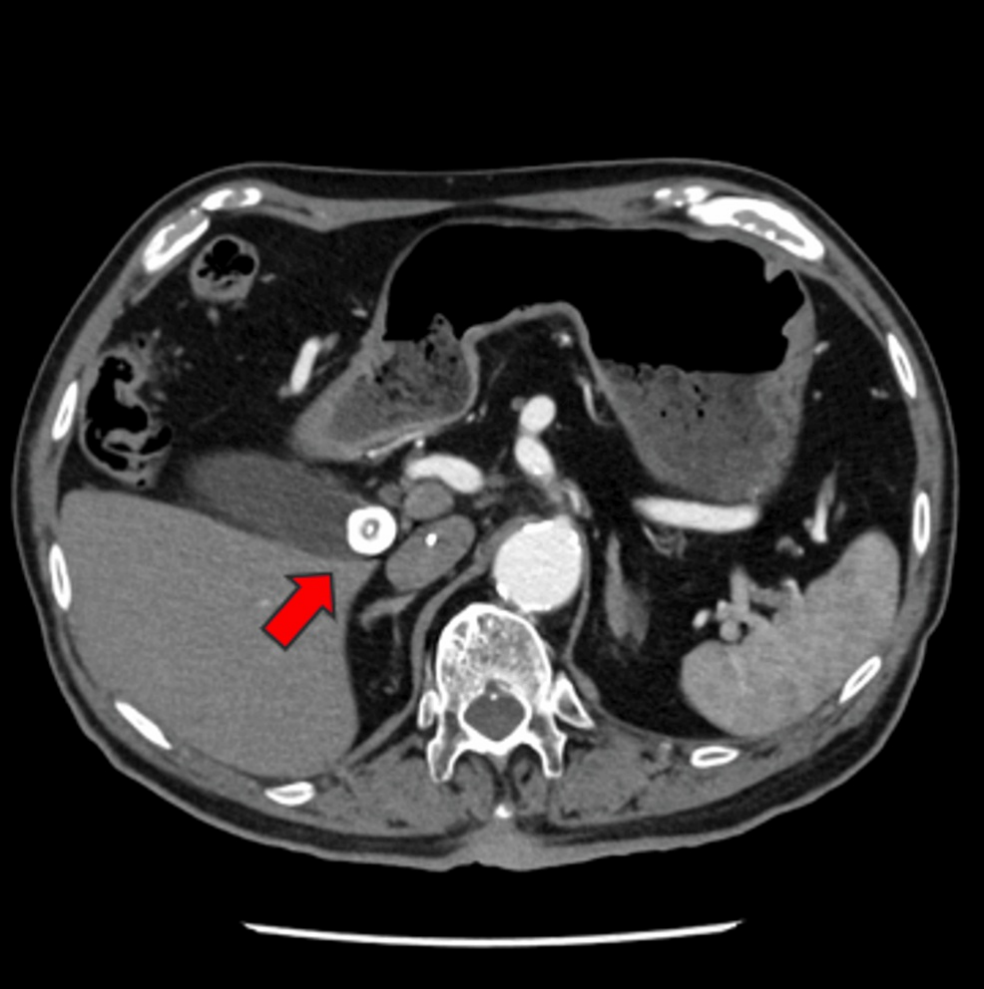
Contrast-enhanced CT performed the day after admission showed gallstones in the gallbladder neck (arrow).
